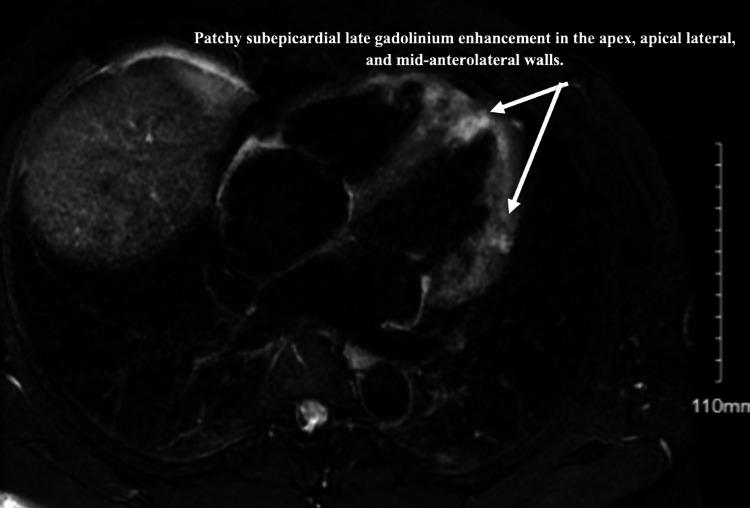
Cardiac MRI - four chambers view showing patchy subepicardial late gadolinium enhancement in the apex, apical lateral, and mid-anterolateral walls. Subendocardial late gadolinium enhancement is evident in the apex as well. MRI: magnetic resonance imaging
